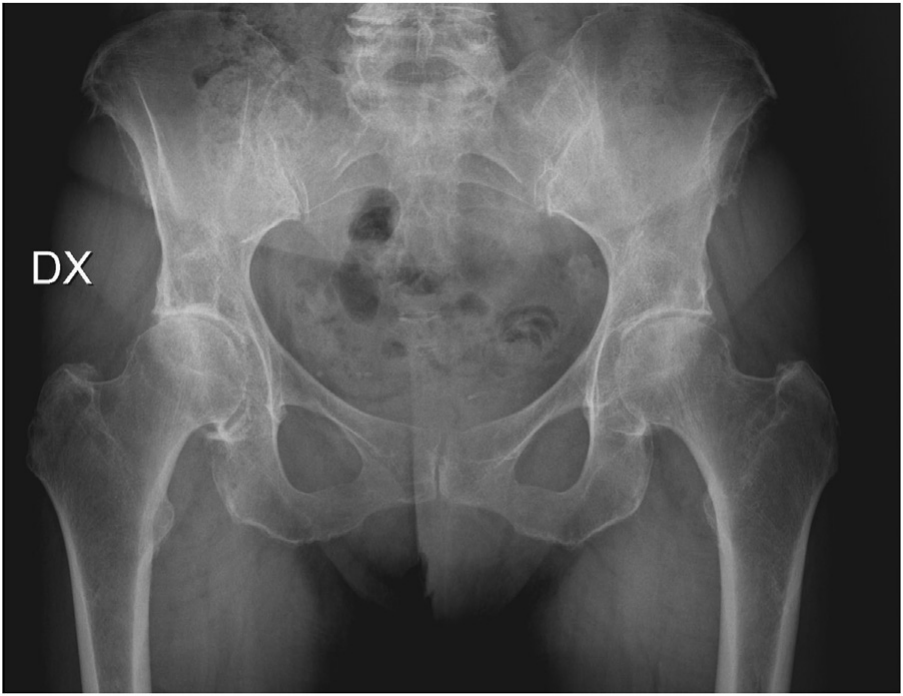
Preoperative x-ray of bilateral hip osteoarthritis in a 67-year-old woman.
